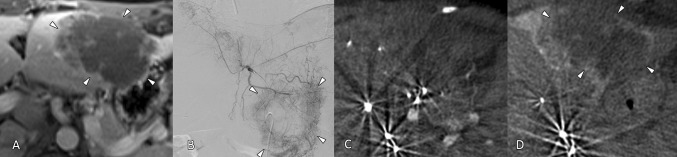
44-year-old woman with a history of colorectal cancer with liver metastases, treated using radio-embolization with Yttrium-90. A Contrast-enhanced T1-weighted gradient-echo sequence in the portal venous phase shows a large, mainly necrotic lesion with rim enhancement in segment 3. B On the DSA images acquired with the microcatheter tip in the left hepatic artery, the lesion is well depicted. C However, on early arterial phase CBCT images, the lesion is not visible. D On delayed arterial phase CBCT images, the entire extent of the lesion is well depicted (*arrowheads*)
